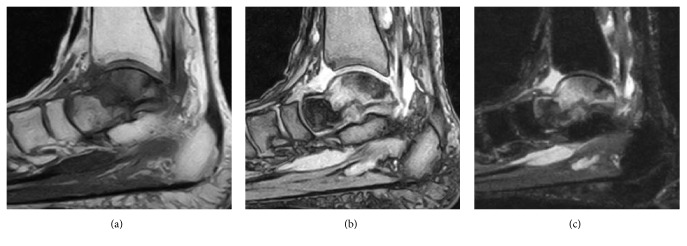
Preoperative MRI scans showing heterogeneous low signal intensity in the talar body on a T1-weighted image (a), low signal intensity in the talar neck and weight-bearing area of the talar body on a T2-weighed image (b), and heterogeneous high signal intensity in the talar body on a STIR image (c).
